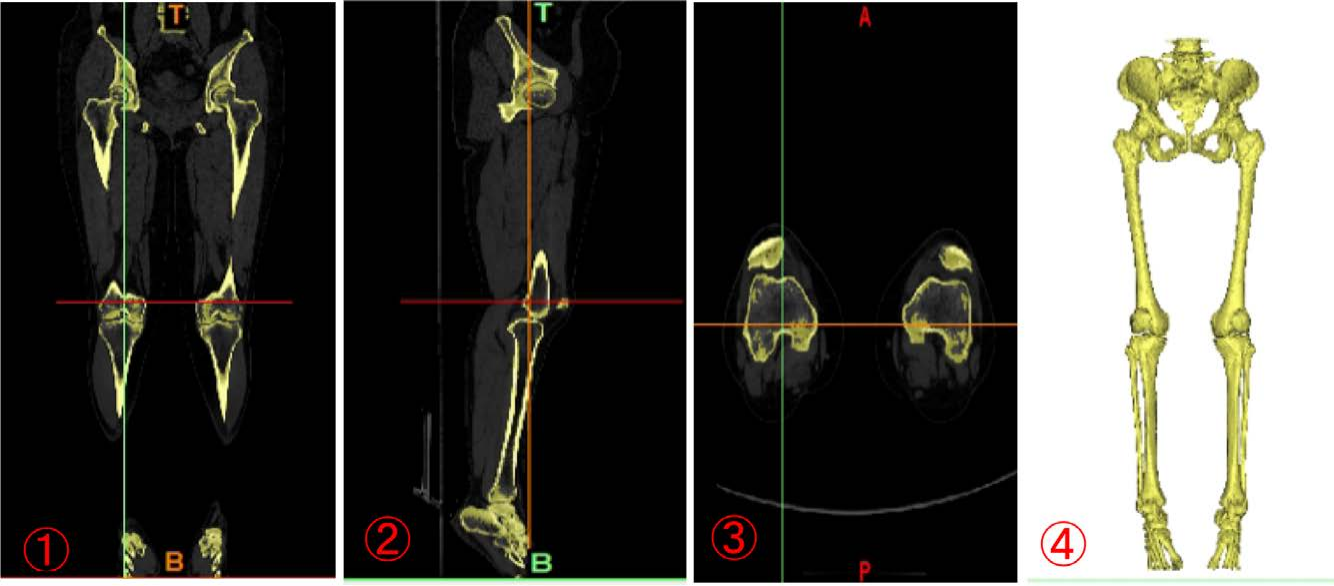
3D CT reconstruction. ①\②\③\④ CT images of both lower limbs in sagittal, coronal, cross-sectional, and 3D processing model, respectively.
